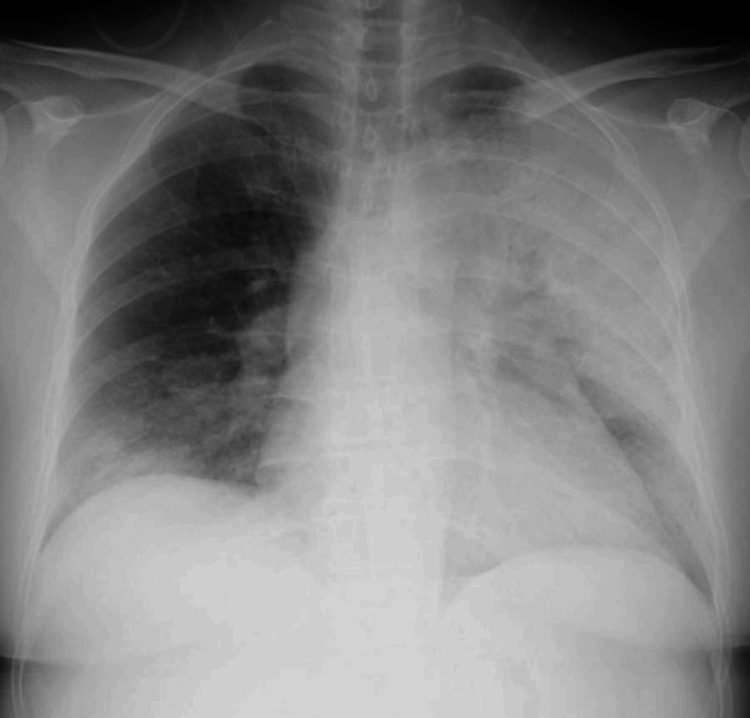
Chest X-ray on admission. Chest X-ray showed an infiltrating shadow in the left lung field.
